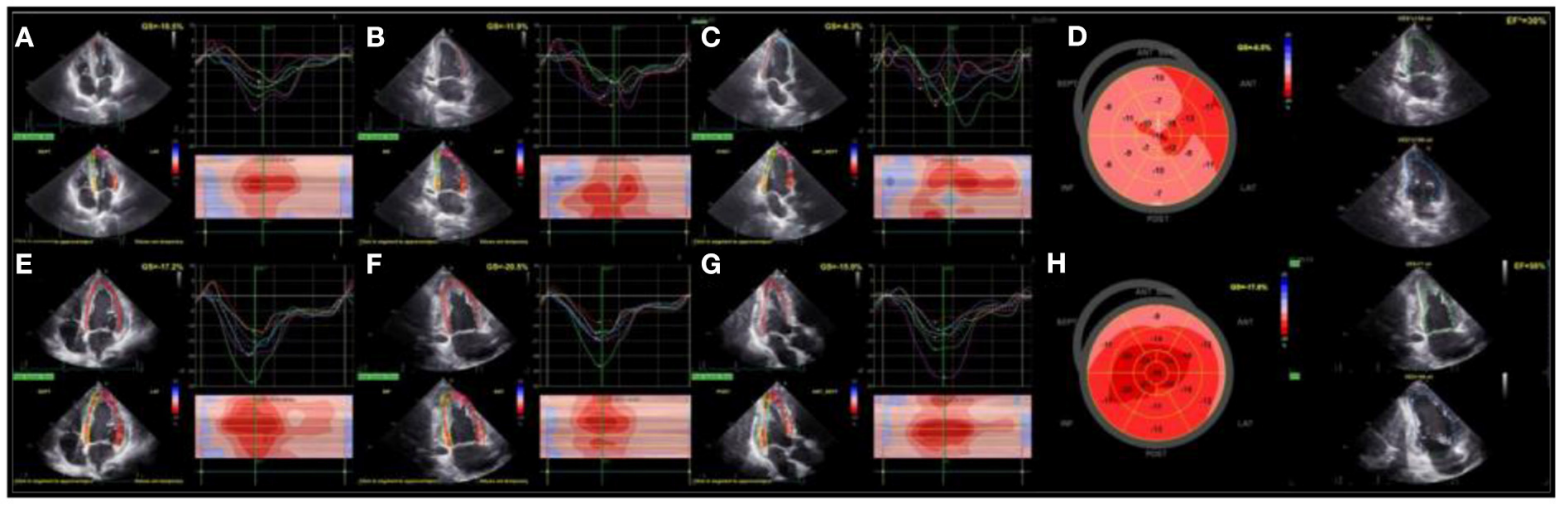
Assessment of LV function with TTE (On the fifth day of admission): LV systolic function was severely impaired, including impaired and asynergic longitudinal deformations that can be appriciated from speckle tracking analysis of apical 4- chamber (A), 2-chamber (B), and 3-chamber (C) views and globally from polar map (D). During the follow-up (eighteenth day), LV EF and longitudinal deformations significantly improved (E–H). LV, left ventricle; TTE, transthoracic echocardiography; EF, left ventricular ejection fraction.
